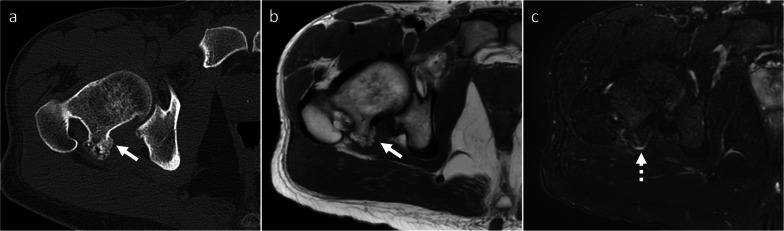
Osteochondroma. On axial CT (a) and T1-weighted MRI (b) images, osteochondroma (white arrow) exhibits cortico-medullary continuity with the underlying native bone. On axial fat-saturated T2-weighted MRI sequence, the lesion shows a thin and uniform cartilaginous cap (dashed arrow). Osteochondroma was pathologically proven
